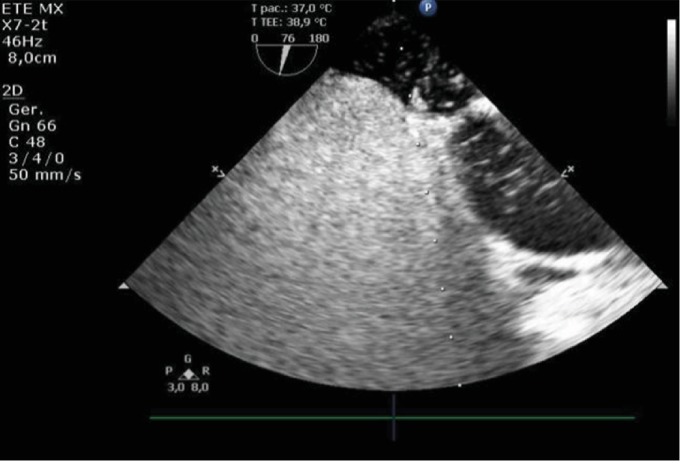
Transesophageal echocardiogram. Image revealing patent foramen ovale. After injection of agitated saline, a right-to-left shunt was observed.
